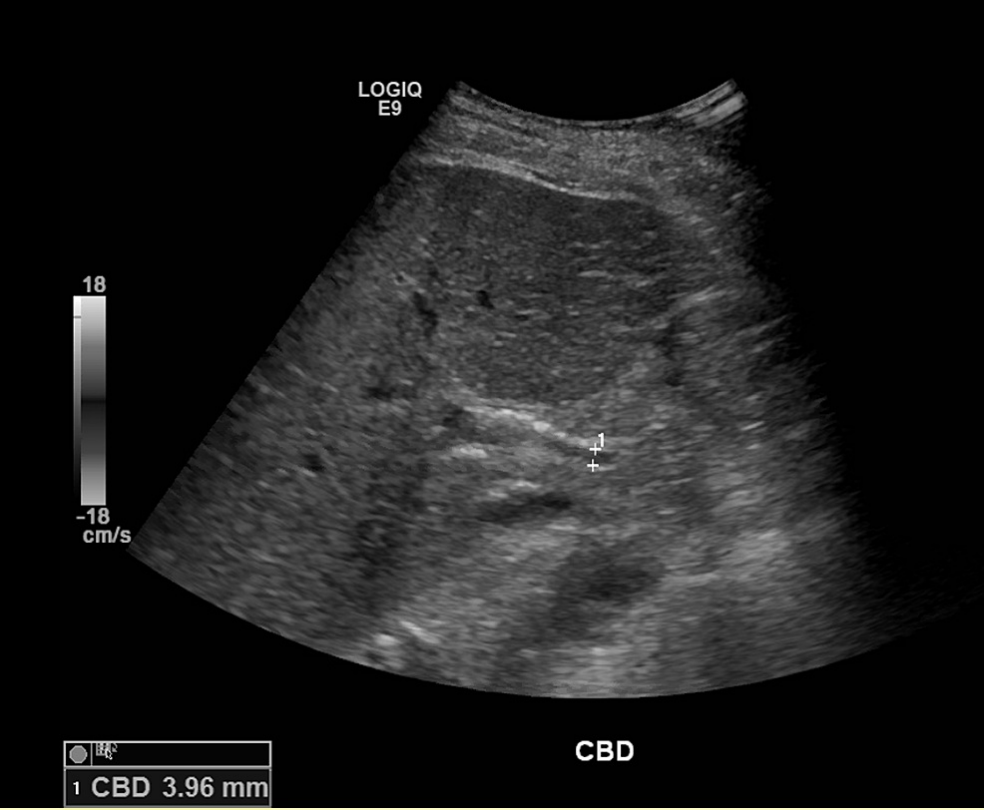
Liver ultrasound showing increased echogenicity of the hepatic parenchyma with no common bile duct dilation. CBD: common bile duct.
